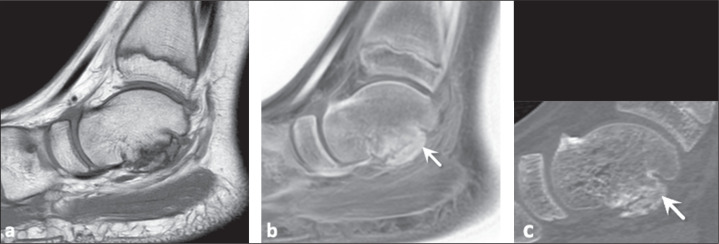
A 12-year-old boy with talocalcaneal coalition. Conventional T1-weighted
